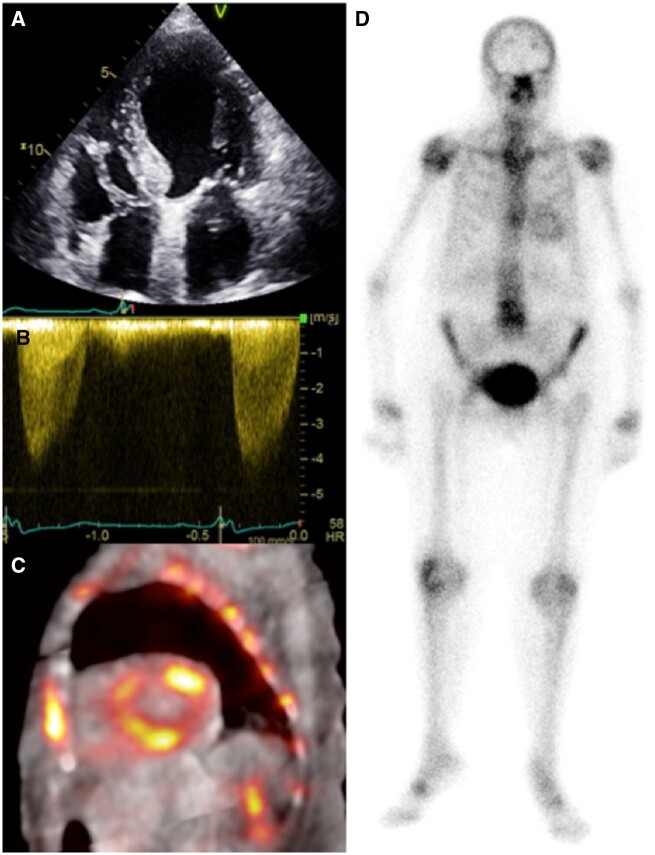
A 90-year-old man with severe aortic stenosis referred for transcatheter aortic valve implantation. Apical four-chamber echocardiography (*A*) shows normal biventricular size with mild left ventricular hypertrophy (1.3 cm basal septum). Continuous-wave Doppler confirmed severe aortic stenosis (peak velocity > 4 m/s) (*B*). The ^99m^Tc-3,3-diphosphono-1,2-propanodicarboxylic acid fused single-photon emission computed tomography/computed tomography short-axis (*C*) confirms diffuse cardiac retention of tracer, visible also on the planar image (*D*), compatible with Perugini grade 2.
